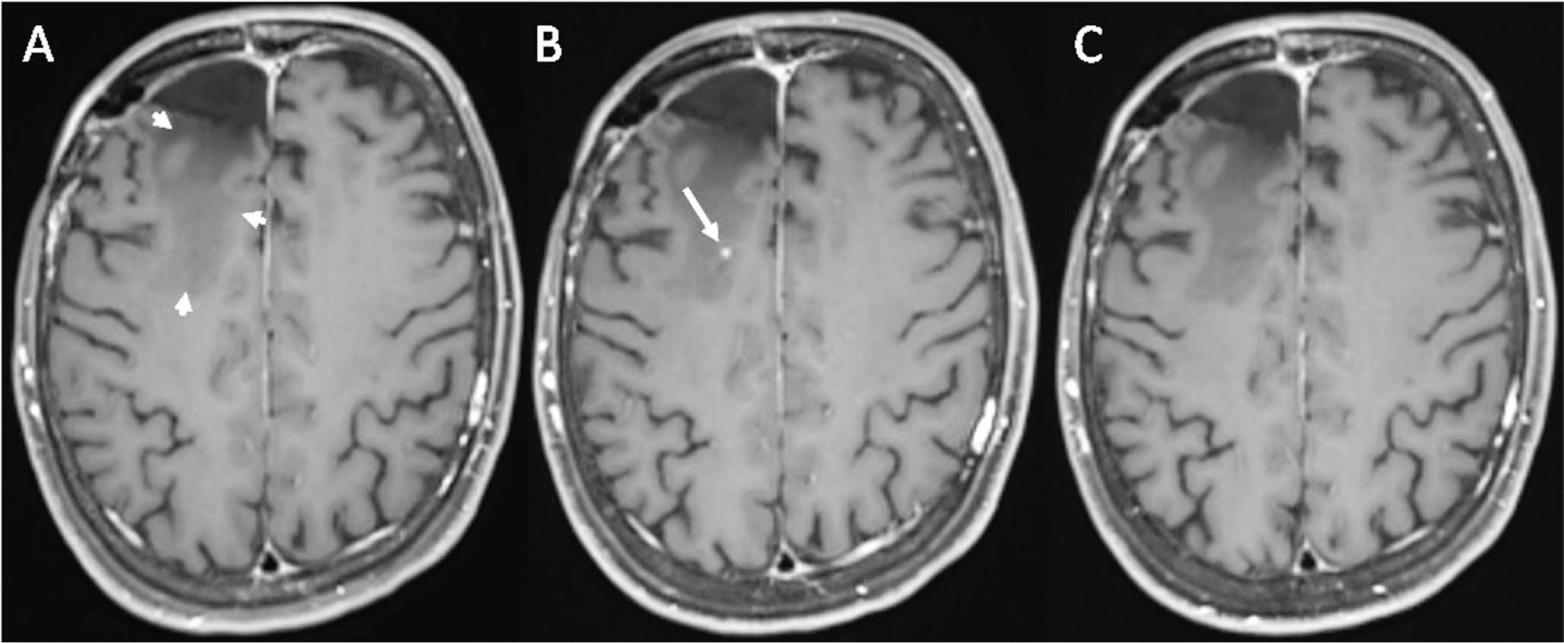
Example of a probable postradiogenic contrast enhancing lesion (pCEL). 49-year old female patient with right frontal oligodendroglioma WHO grade III, who received tumor resection followed by proton radiotherapy. Follow up MRI performed 2years after end of radiation therapy (A) shows post-therapeutic leucencephalopathy (arrow heads) in the right superior frontal gyrus. Next available follow up MRI, performed 5 month later (B) depicts a new, dot-like parenchymal contrast enhancement (arrow). This lesion was present on two further follow-ups (not shown), but was no longer depictable on MRI performed 12 months after its initial detection (C), and therefore fullfilled the imaging criteria of a probable postradiogenic contrast-enhancing lesion. A-C:Axial reconstructions of the 3D-T1-weighted sequence after intravenous contrast application
